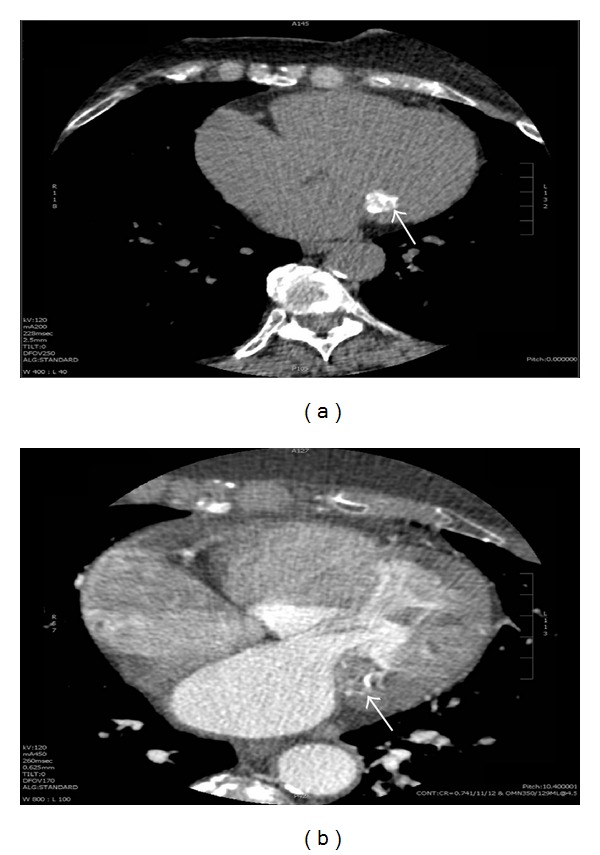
Chest CT scan without and with contrast. A 17 × 18 mm round hyperdense mass was identified (white arrow) along the mitral valve annulus (a). There is a heterogeneous calcification pattern with a hypoattenuated necrotic center (b).
